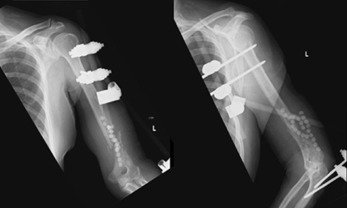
Post-operative radiographs with external fixator and antibiotic beads.
